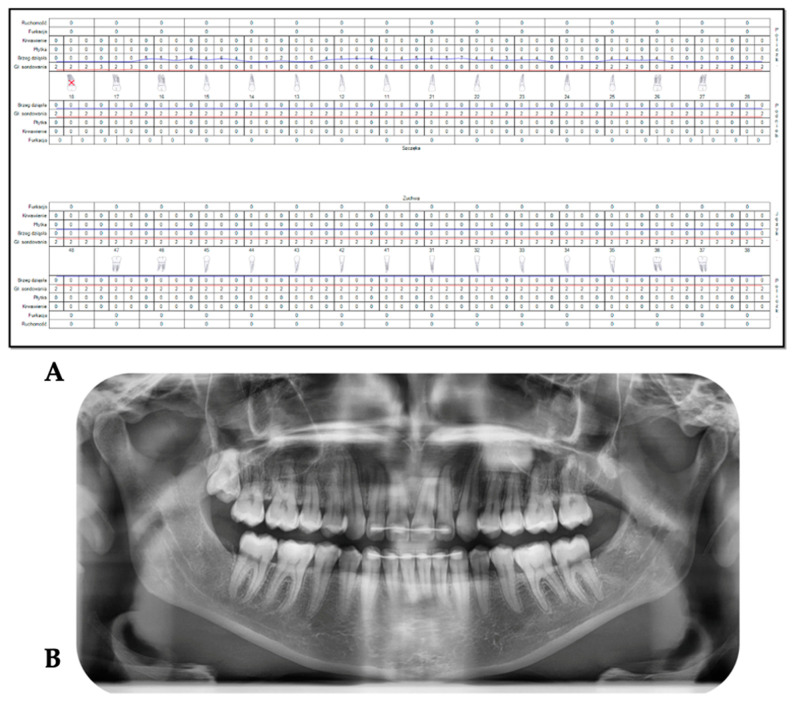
(A,B). Pre-operative clinical and radiographic investigations. Photo (A) (top) is the periodontal charting, showing no evidence of any abnormality. Photo (B) (bottom) shows no evidence of any hard tissue abnormalities on the orthopantomogram.
